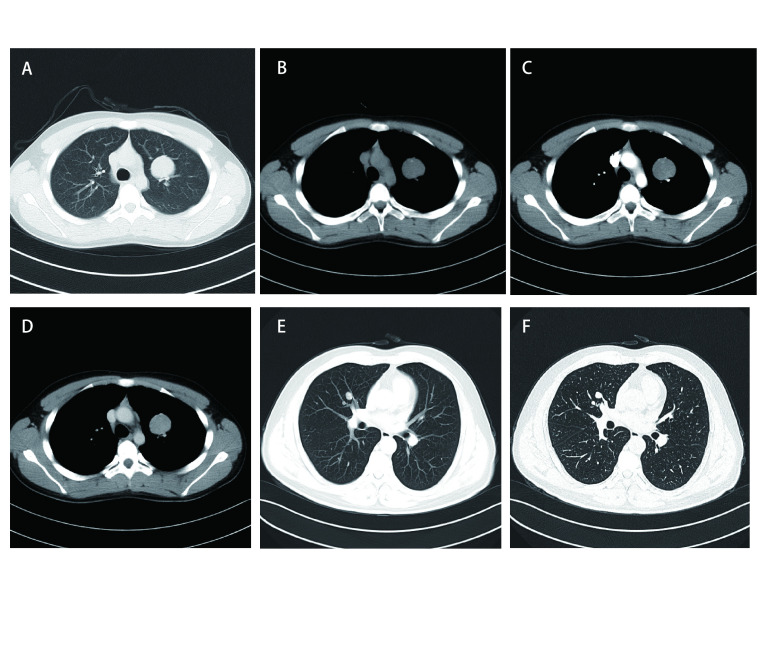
胸部CT：病灶典型。A、B、C、D为同一患者：左肺上叶见一类圆形肿块，边界清晰，内见点状钙化影，增强CT扫描均匀强化；E、F为同一患者（F为薄层CT）：右肺中叶见两个小结节，边界清楚，边缘见点状钙化。 Chest CT: lesions are typical. A, B, C and D are the same patient: A round mass is seen in the left upper lobe, with a clear boundary, punctate calcifications are seen inside, and the enhanced scan is evenly enhanced; E and F are the same patient (F is thin-slice CT): Two small nodules with clear boundaries and spotted calcifications on the edges are seen in the right middle lobe.
